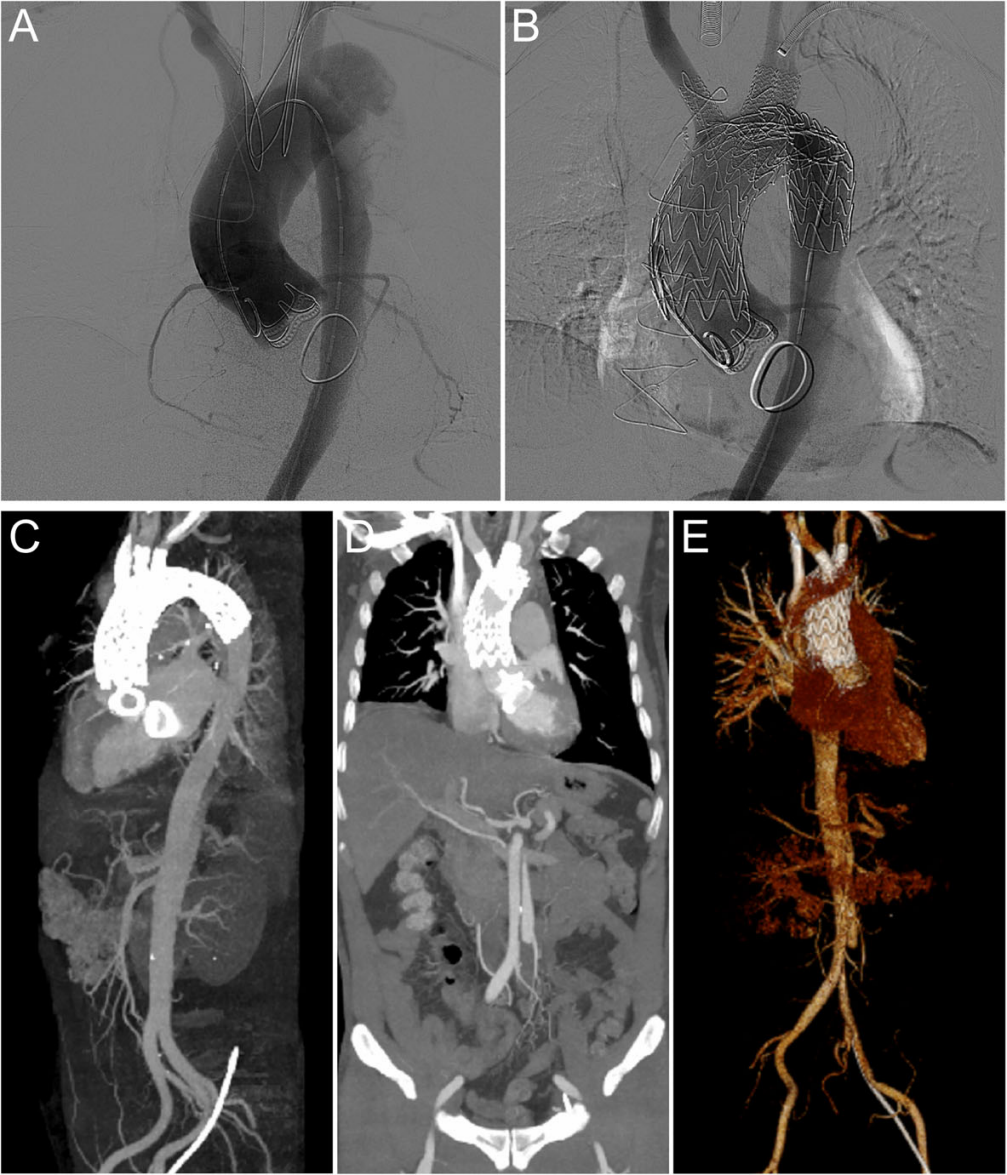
In situ laser fenestration for revascularization of aortic arch during treatment for iatrogenic type A aortic dissection. (a) Aortography was performed via the left femoral artery to measure the diameters of the ascending aorta and arch vessels; (b) Aortography was performed after stent implantation demonstrated no endoleak, and aneurysm and dissection; (c-e) CTA presented possible access sites of fenestration, stent placement, patency of the aortic arch branches. CTA, computed tomographic angiography
